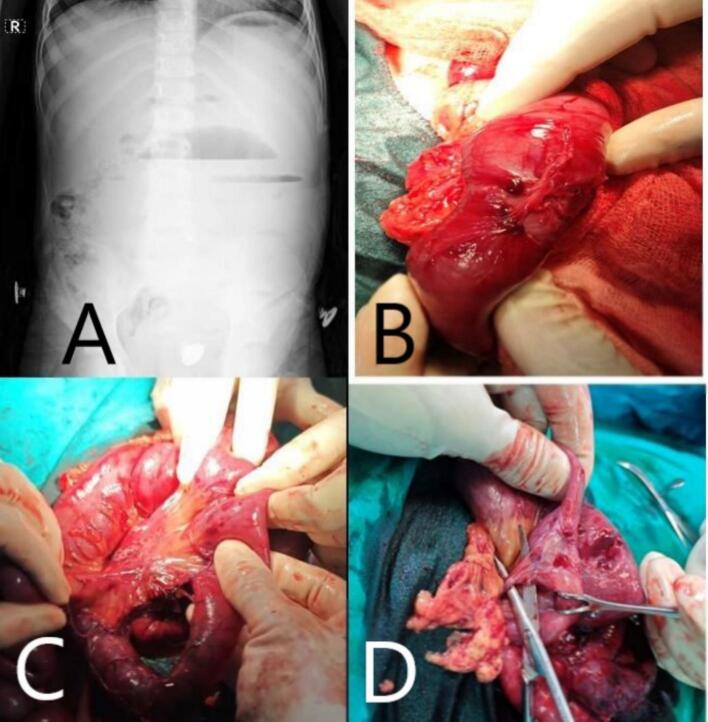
Radiological and intraoperative findings. (A): Plain abdominal X-ray showing multiple air-fluid levels, dilated bowel loops and an absence of distal gas suggestive of small bowel obstruction. (B): Intraoperative view demonstrating dilated, proximal small bowel with an abrupt transition point resulting by adhesions. (C): Intraoperative view demonstrating distended proximal segment adjacent to a collapsed distal bowel; and mesenteric injury. (D): Intraoperative view demonstrating bands narrowing the bowel.
